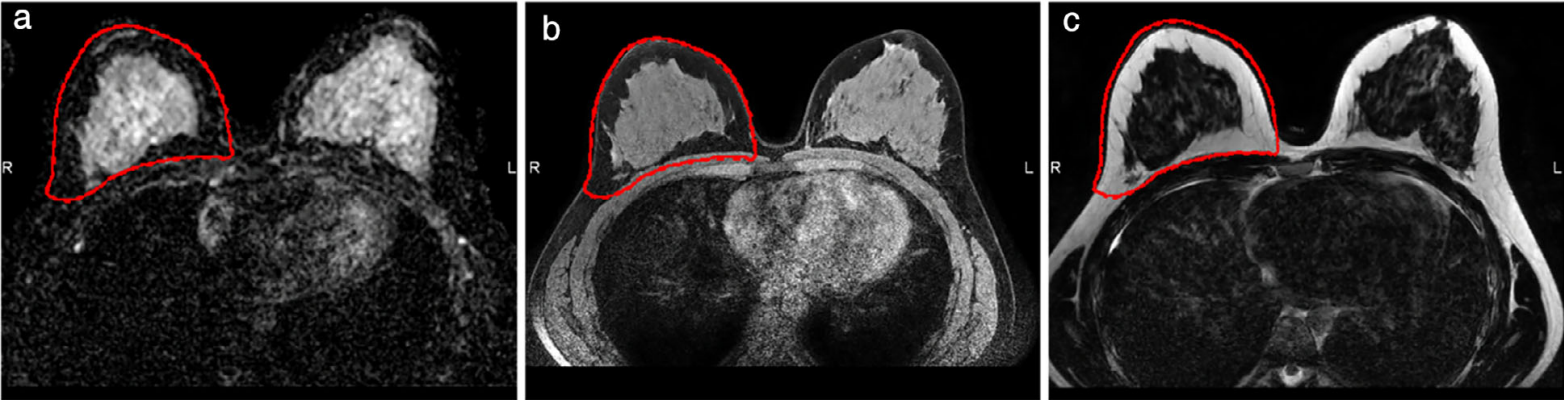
An axial slice of a 3D MRI exam of a healthy volunteer including right breast segmentation (red margin). (a) ADC map, (b) T2‐weighted image, (c) T1‐weighted image
